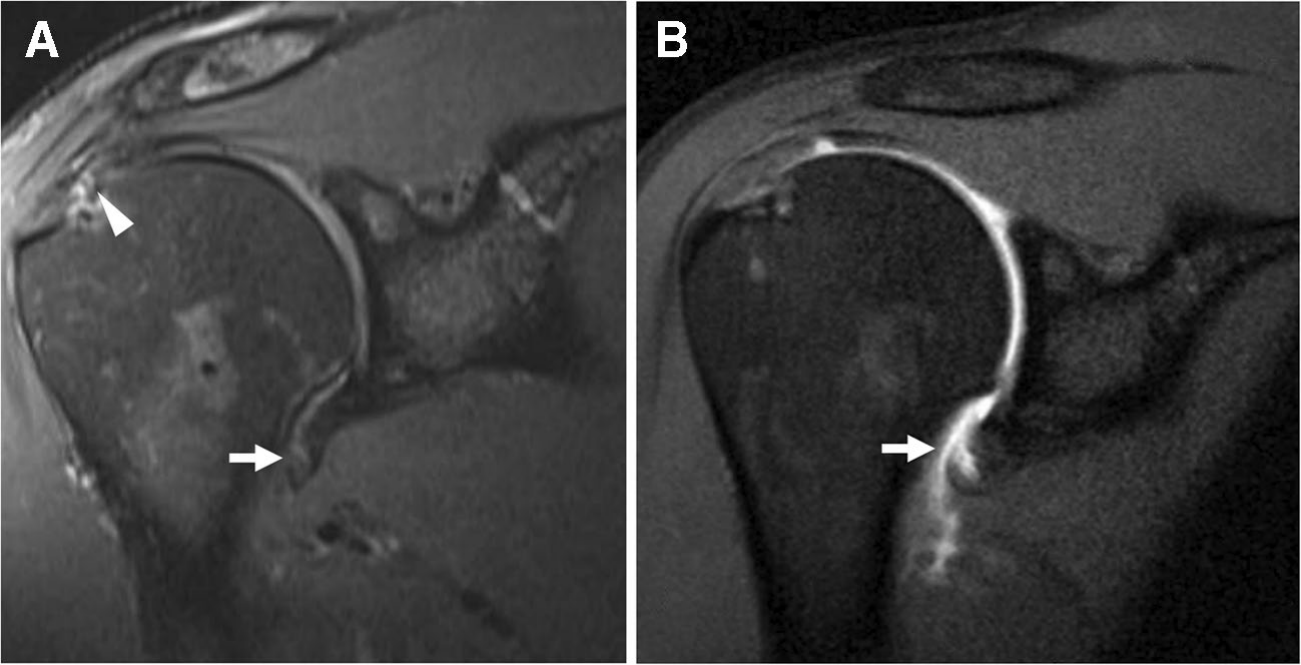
Chronic humeral avulsion of the glenohumeral ligament (HAGL). Twenty-seven-year-old professional pitcher with shoulder pain. A Coronal intermediate-weighted fat-suppressed conventional 1.5 T MR image shows irregularity of the inferior glenohumeral ligament (IGHL) complex (arrow) and partial-thickness tearing of the supraspinatus tendon (arrowhead). B Coronal T1-weighted fat-suppressed 1.5 T MR arthrogram image shows extra-articular contrast leakage and a thickened, retracted IGHL margin, consistent with a HAGL
